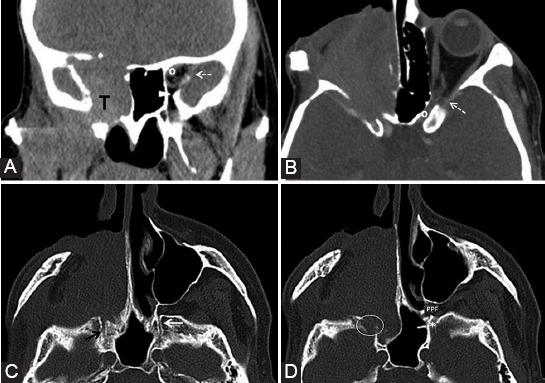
Second relapse of a neuroblastoma (T) of the right nasal fossa and maxillary sinus. Coronal reformatted computed tomography (CT) (A) and axial CT with contrast medium (B) images show complete invasion of the optic canal (o), superior (dashed arrow) and inferior orbital fissure (arrowhead) on the right compared to the normal left side (indicators for the normal left side). Axial CT scan (C, D, bone window) shows enlarged right vidian canal with slight blurring of its contour (black arrow) as compared to the left (white arrow). The right foramen rotundum (ellipse) is eroded compared to the left side (*). Notice the normal fat pad in the left pterygopalatine fossa (PPF) and total obliteration on the right.
